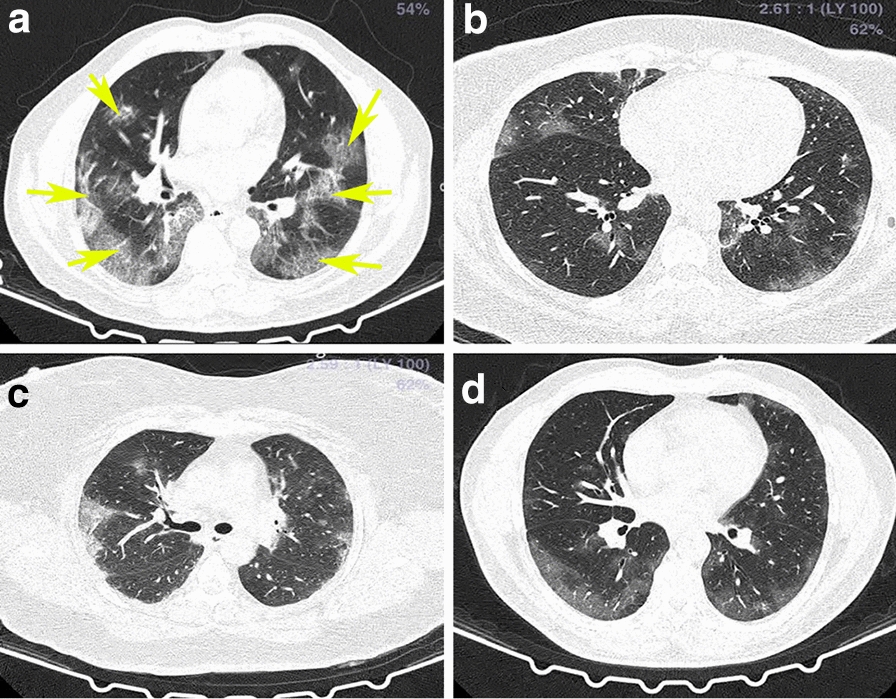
a–d Representative CT scans of a COVID-19 patient. Yellow arrows show ground glass opacities
